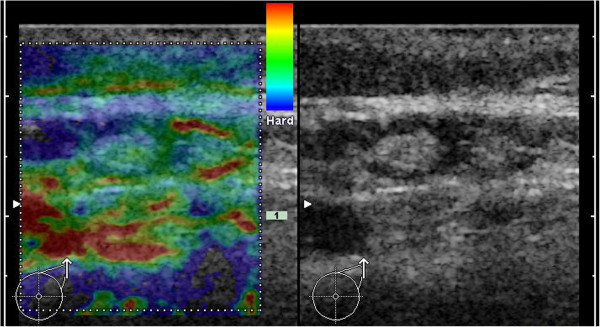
Example for B-mode ultrasound and elastogram of a healthy LN. In B-mode ultrasound the LN exhibits no criteria for malignancy. The predominant color of the medulla is turquoise (with smaller areas of green) and the cortex is mainly green. Applying the criterion of a blue cortex, this case would be a true-negative.
